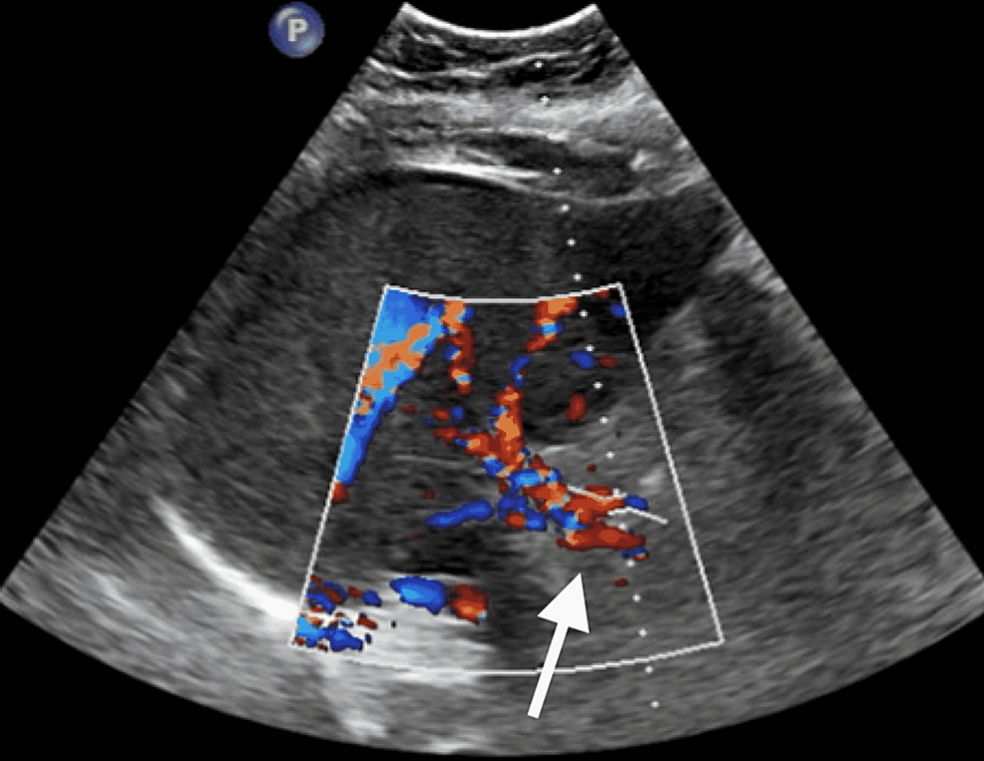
Liver US showing grossly patent main portal vein flow US: ultrasound
